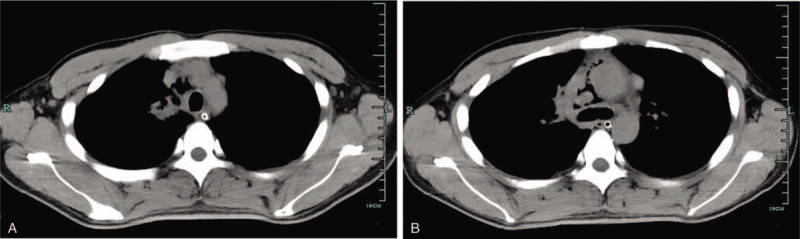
Thoracic computed tomography showing pneumopericardium, pneumomediastinum, and cavernous communicated lesions adjacent to the right superior mediastinum.
